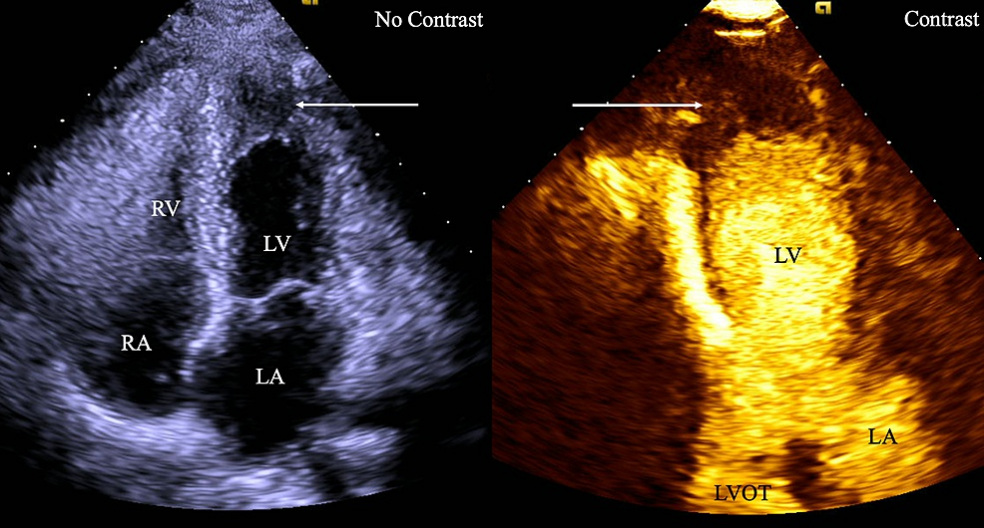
Apical four-chamber view (left) and apical three-chamber view with definity contrast (right) on echocardiography depicting apical hypertrophy and LV thrombus. RV - right ventricle, RA - right atrium, LV - left ventricle, LA - left atrium, LVOT - left ventricular outflow tract
